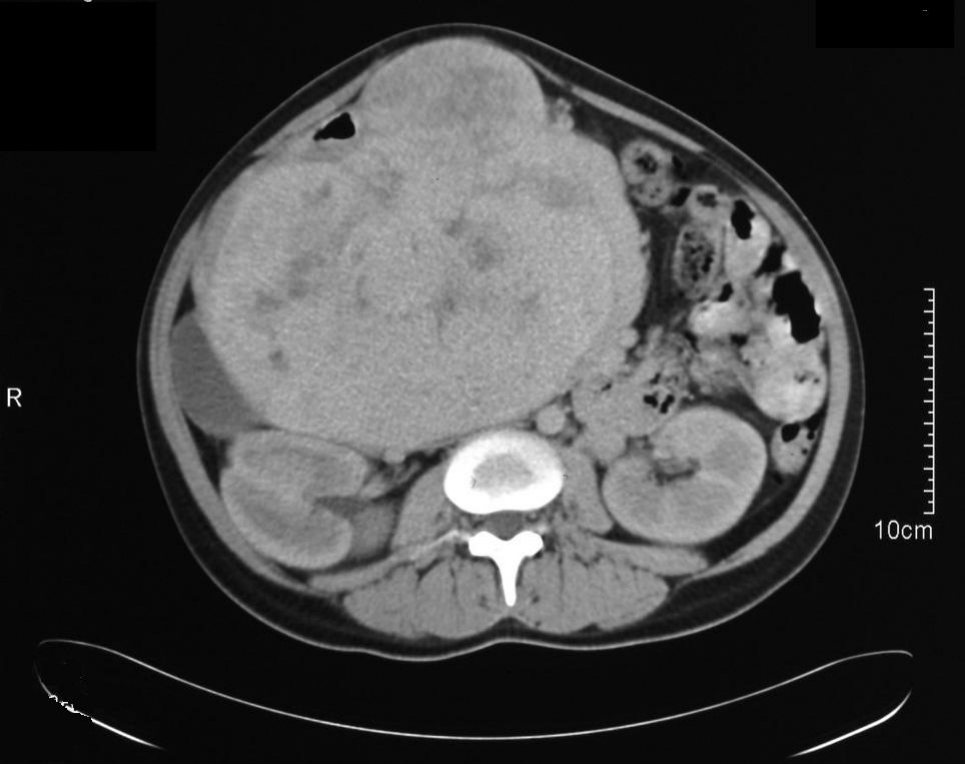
Retropancreatic tumor. A preoperative CT scan showing the large retropancreatic tumor.
